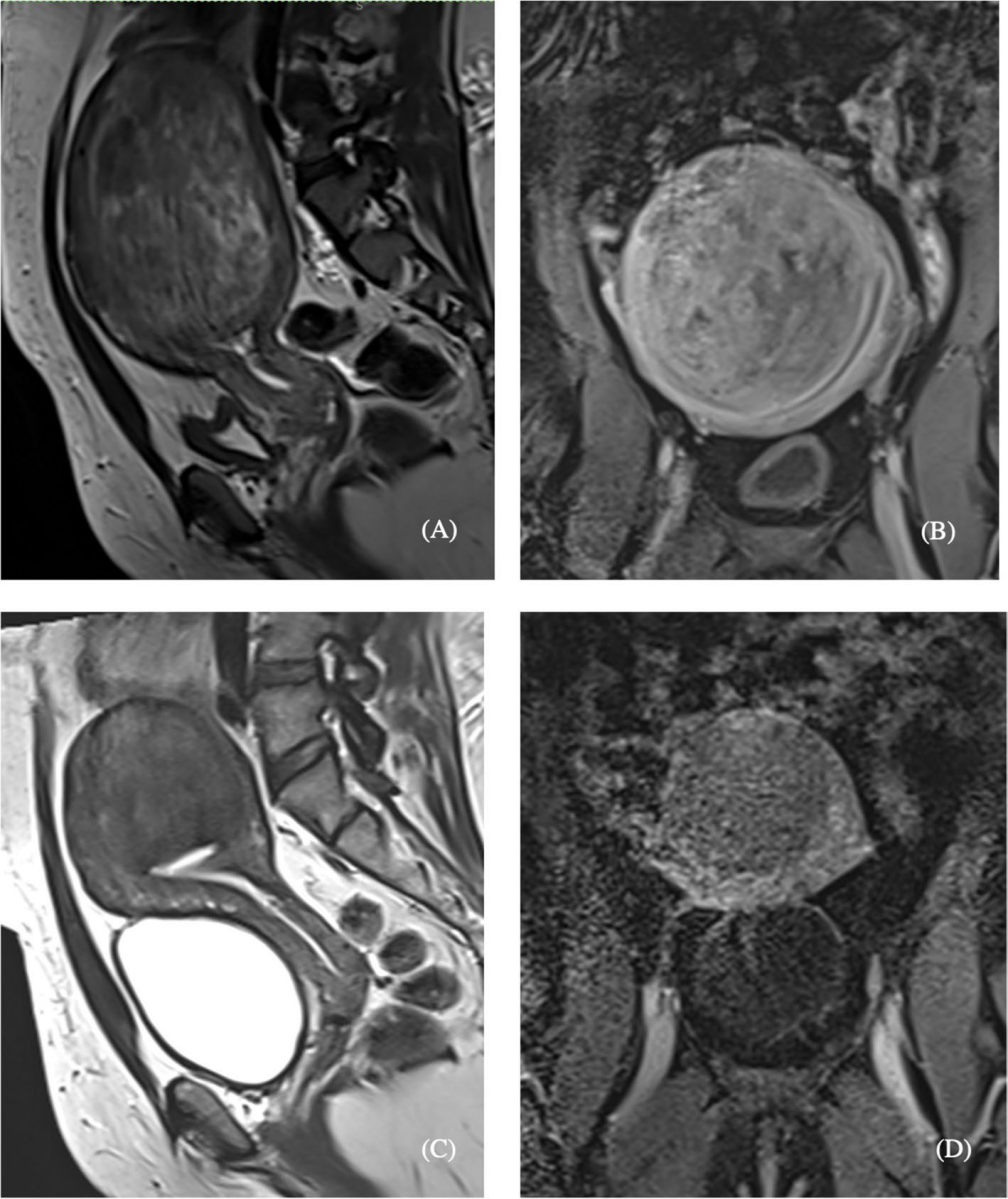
Pelvic MRI demonstrating a uterine fibroid measuring 12.3 × 7.2 × 11.5 cm (530 cm^3^) pre-procedure (A & B) and 6.8 × 6.0 × 7.6 cm (161 cm^3^) 6 months post-procedure (C&D). A & C Sagittal T2-weighted post-gadolinium. B & D Coronal T1-weighted post-gadolinium
